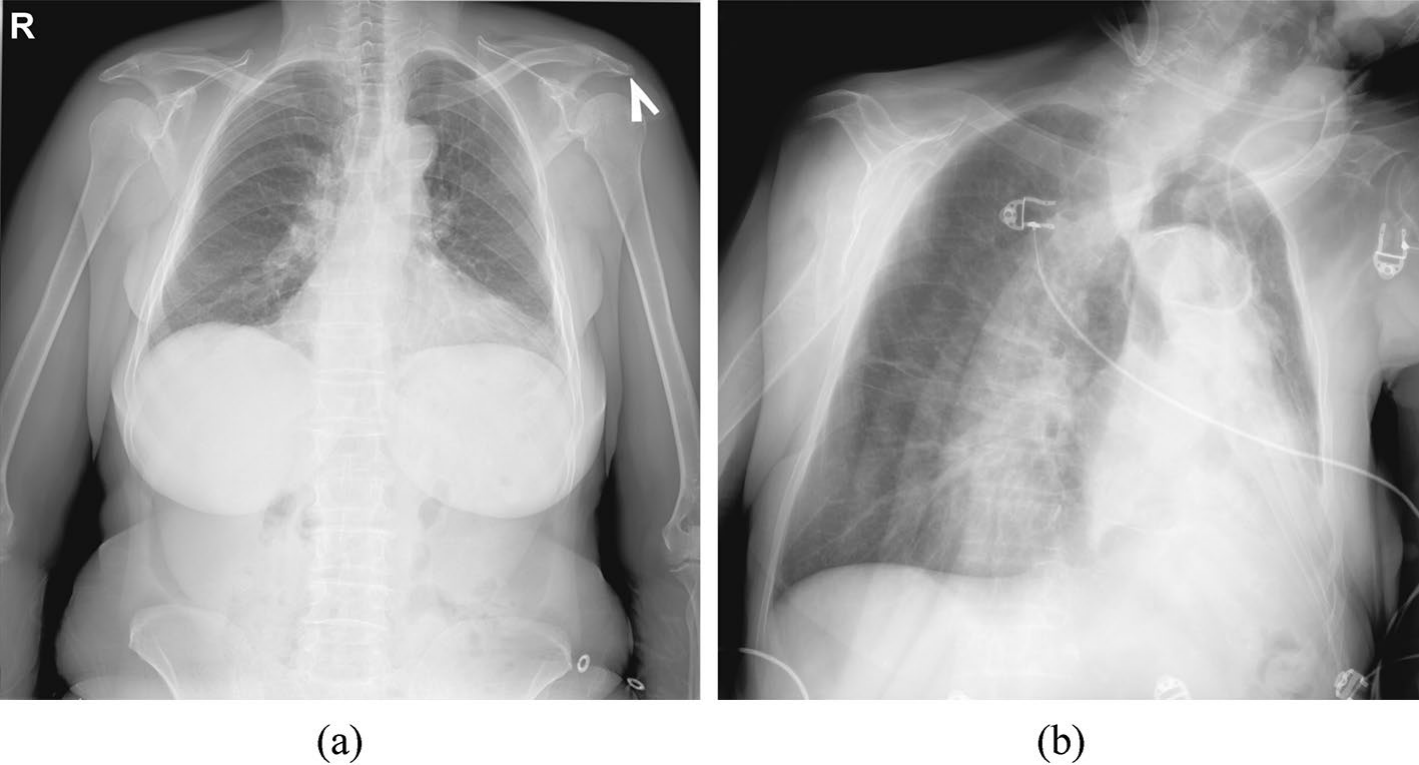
(a) A chest X-ray example, where the inclusion of gender prediction head of the framework corrected a false-positive disease prediction. (b) A chest X-ray example mis-classified as defected, i.e. not representing a fronal chest X-ray.
